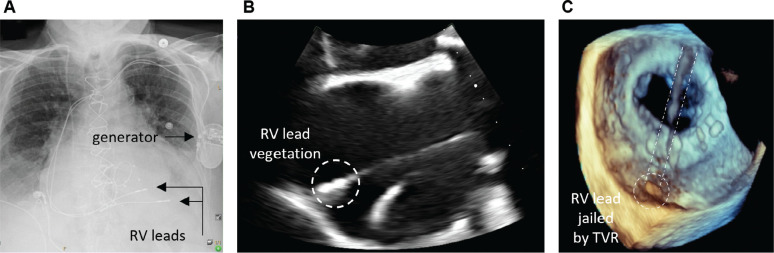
A: A postero-anterior chest X-ray demonstrating a permanent pacemaker generator with two right ventricular (RV) leads, one previously abandoned and the other jailed after a bioprosthetic tricuspid valve replacement. B: A two-dimensional transesophageal echocardiogram demonstrating an RV lead vegetation. C: A three-dimensional transesophageal echocardiogram demonstrating the RV lead entrapped “jailed” by the bioprosthetic tricuspid valve. *Abbreviations:* RV, right ventricle/ventricular; TVR, tricuspid valve replacement.
